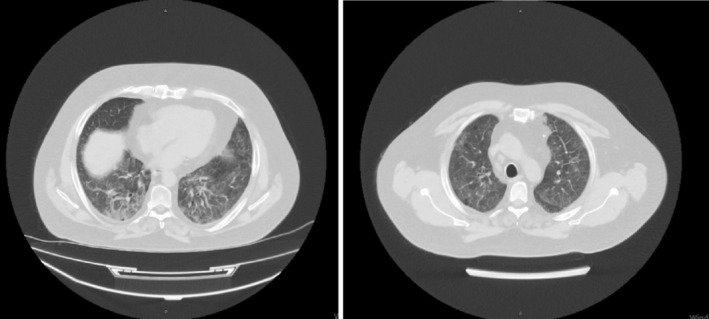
Computed tomography scans showing widespread ground‐glass opacities, septal thickening, fibro atelectatic changes and traction bronchiectasis.
